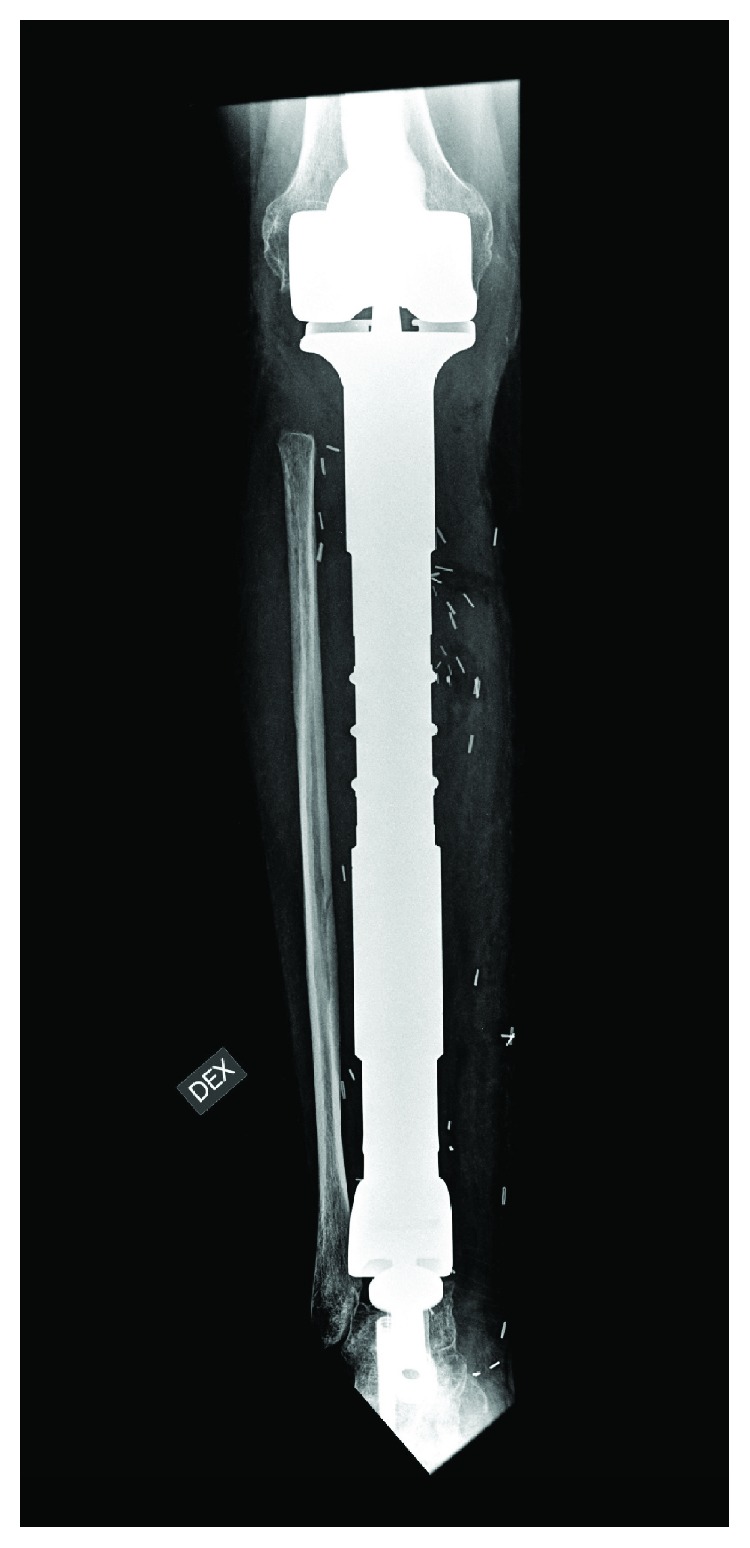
Postoperative X-ray from a right tibia. The knee joint was reconstructed with a metal-on-poly articulation with a (unique) metal-on-metal hinge mechanism, and the ankle joint was reconstructed with a metal-on-poly hinge joint with a talar replacement, stabilized with a trans-talar and trans-calcanear hydroxyapatite-coated stem. A supplementary screw was used to add stability in the subtalar joint.
